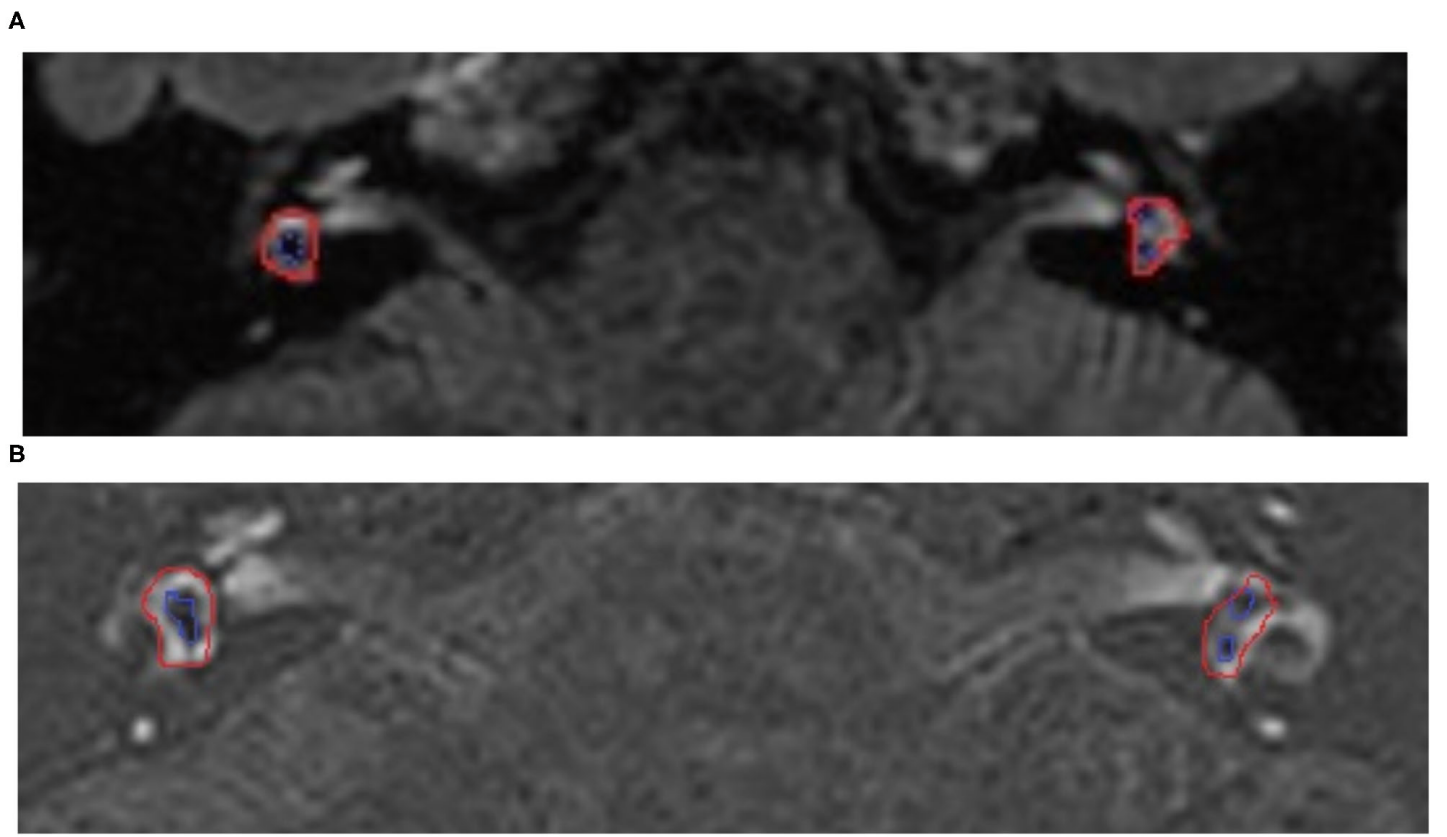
The 3D-fluid-attenuated inversion recovery (FLAIR) MRI views of a 50-year-old woman with Meniere's disease (MD) on the right side before and after surgery. The long-term control of vertigo 2 years after the operation was class A. (A) MRI before the operation, the red line indicated the entire vestibular area, while the blue line indicated the endolymphatic area. The low density of the vestibular on the right was larger than the left, showing vestibular hydrops on the right side. (B) MRI after the operation, the low density of the vestibular on the right was still larger than the left, but did not vary after surgery. This result indicated the vestibular hydrops was not alleviated before and after surgery.
